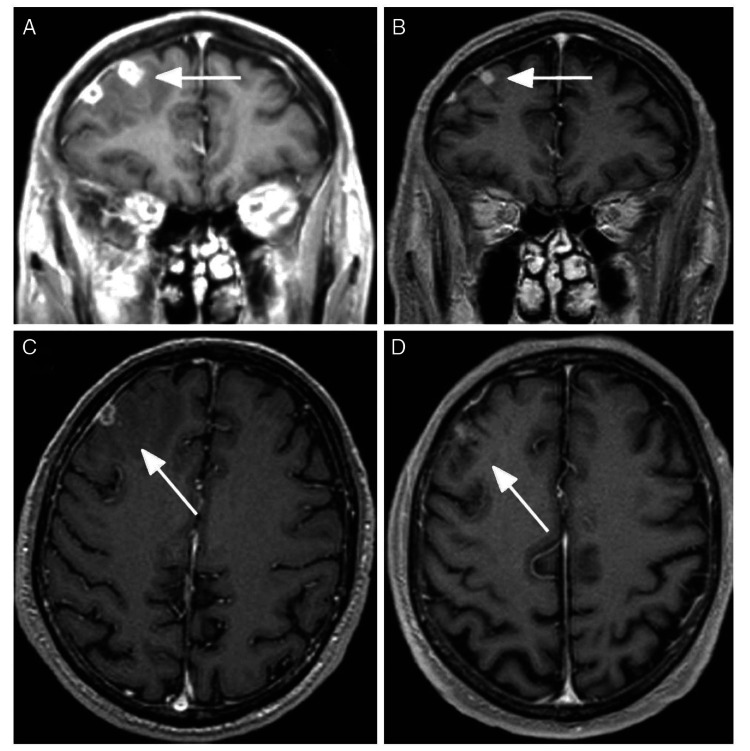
Enhanced brain MRI before and after treatment. Contrast-enhanced brain MRI of patient with tuberculoma in right frontal area with perilesional edema. A. Coronal image before treatment; B. Coronal image three months after treatment showing reduced size of the lesions; C. Axial image before treatment; D. Axial image three months after treatment [Courtesy Bessolo E, Villate S, Arroyo JA, Rango G, Ortiz GA. Tuberculoma cerebral en placa. Presentacion atipica de tuberculosis en el sistema nervioso central. Neurol Arg 2017;9(3):195-198].
